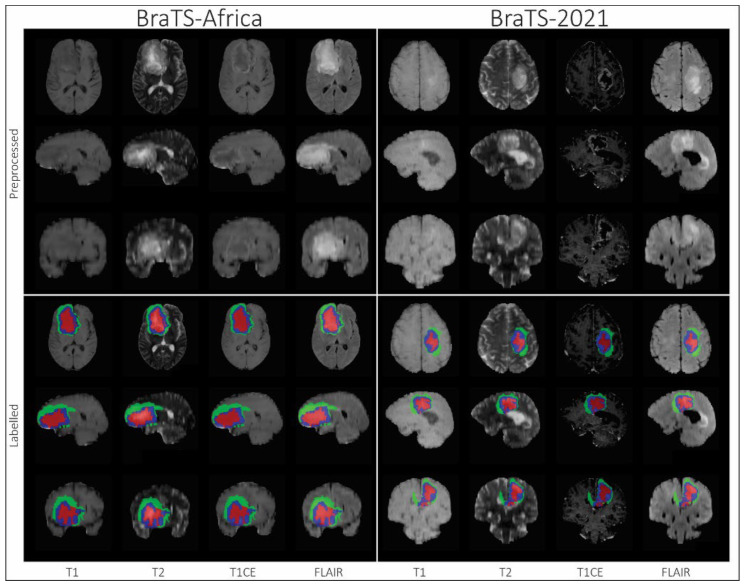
Typical clinical brain MRI obtained in Sub-Saharan Africa (left) compared to a representative case from the 2021 BraTS challenge (right). The images display notably lower resolution—especially in the sagittal plane—when compared to standard clinical imaging from high-income regions. These variations in image quality and acquisition parameters can pose additional challenges for automated algorithms. Figure adapted from [25], licensed under CC BY 4.0: https://creativecommons.org/licenses/by/4.0/ (accessed on 25 September 2024).
